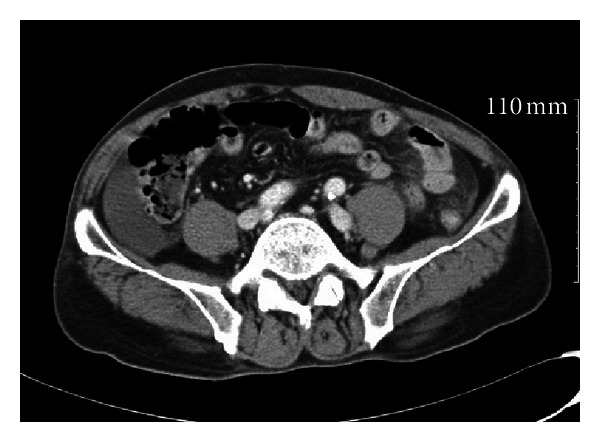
Abdominal computed tomography (CT) revealed fluid accumulation in the paracolic gutter on the right side.
